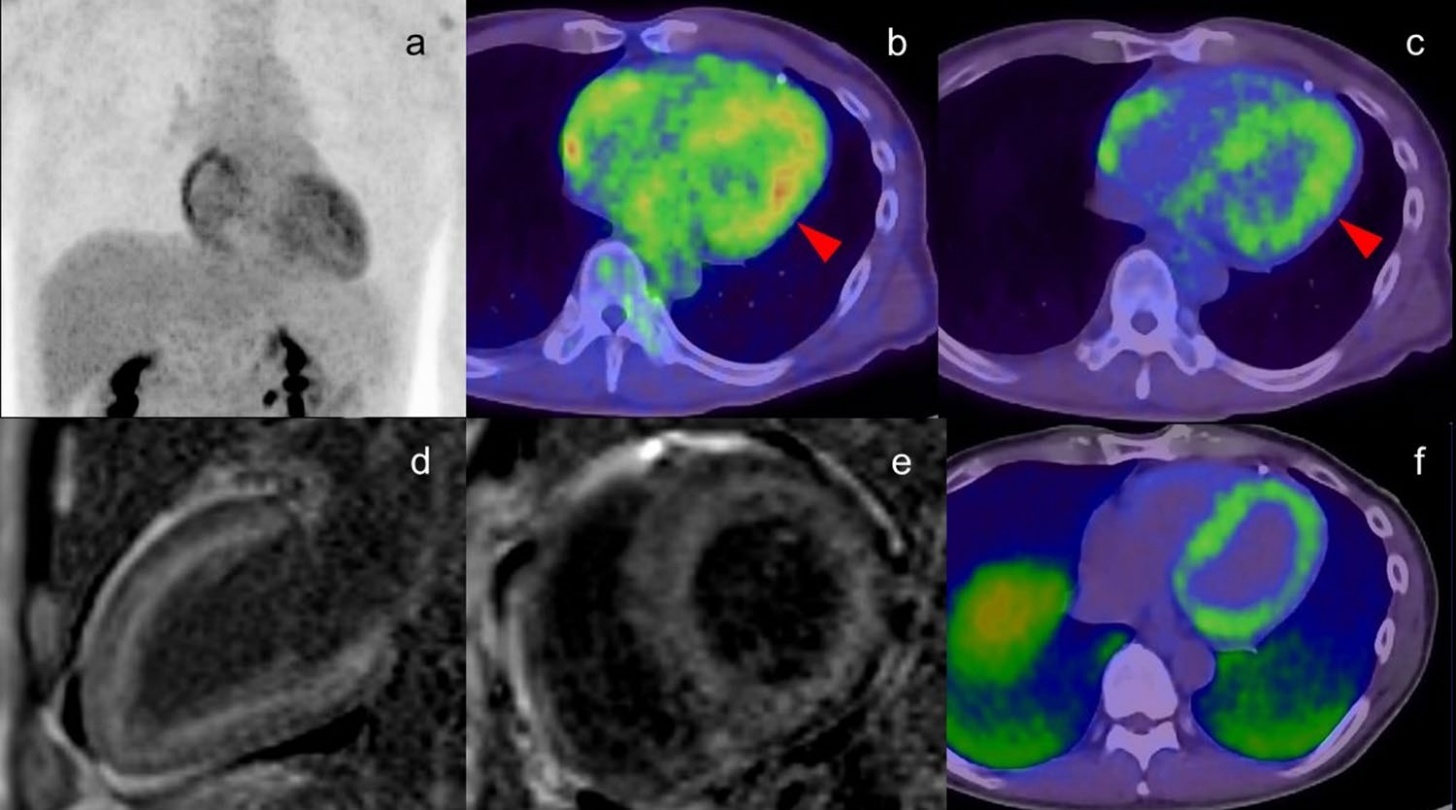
Cardiac amyloidosis. Moderate FDG uptake was confirmed in left ventricle wall in the patient with AL amyloidosis (a, b red arrowhead). Different color scale of FDG PET/CT imaging demonstrated that FDG uptake existed in inner layer of left ventricle wall, which were matched to the area with high intensity in PSIR MRI image (d, e) and the uptake in PiB PET/CT imaging (f) suggesting the amyloid deposit. The FDG uptake in the right atrium was cause by overload pressure of right atrium
